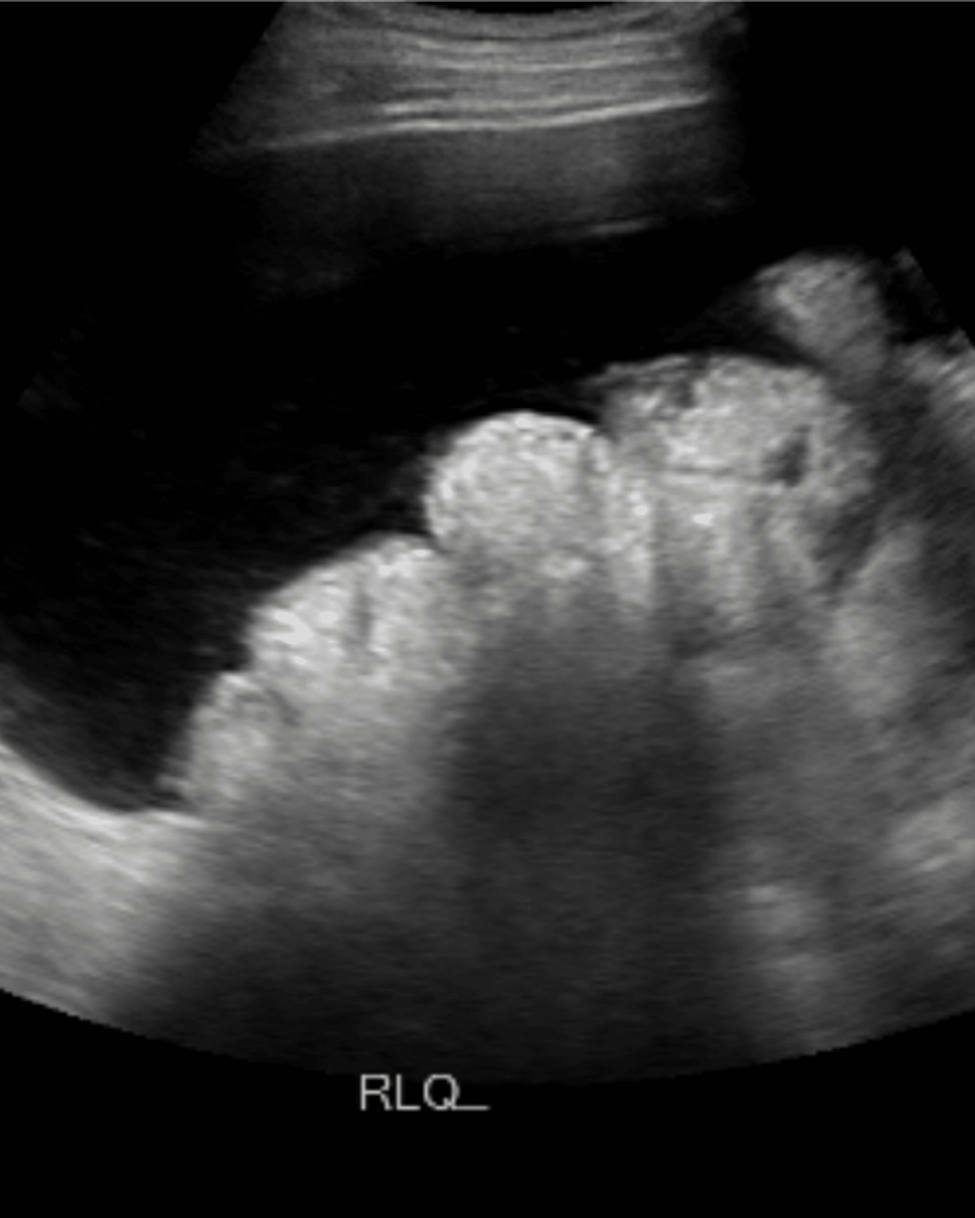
Ultrasound Paracentesis finding of a total of 5500cc of chylous ascites prior to removal.
